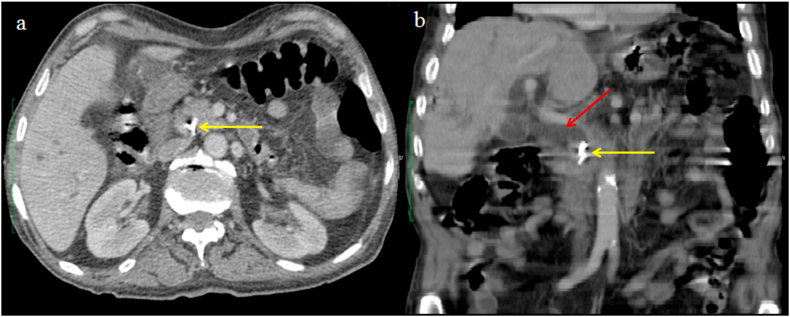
Contrast-enhanced computed tomography showing a surgical clip in the distal CBD (yellow arrow) associated with widened CBD up to 1,5 cm (red arrow) on axial (a) and coronal (b) sections. (For interpretation of the references to colour in this figure legend, the reader is referred to the Web version of this article.)
